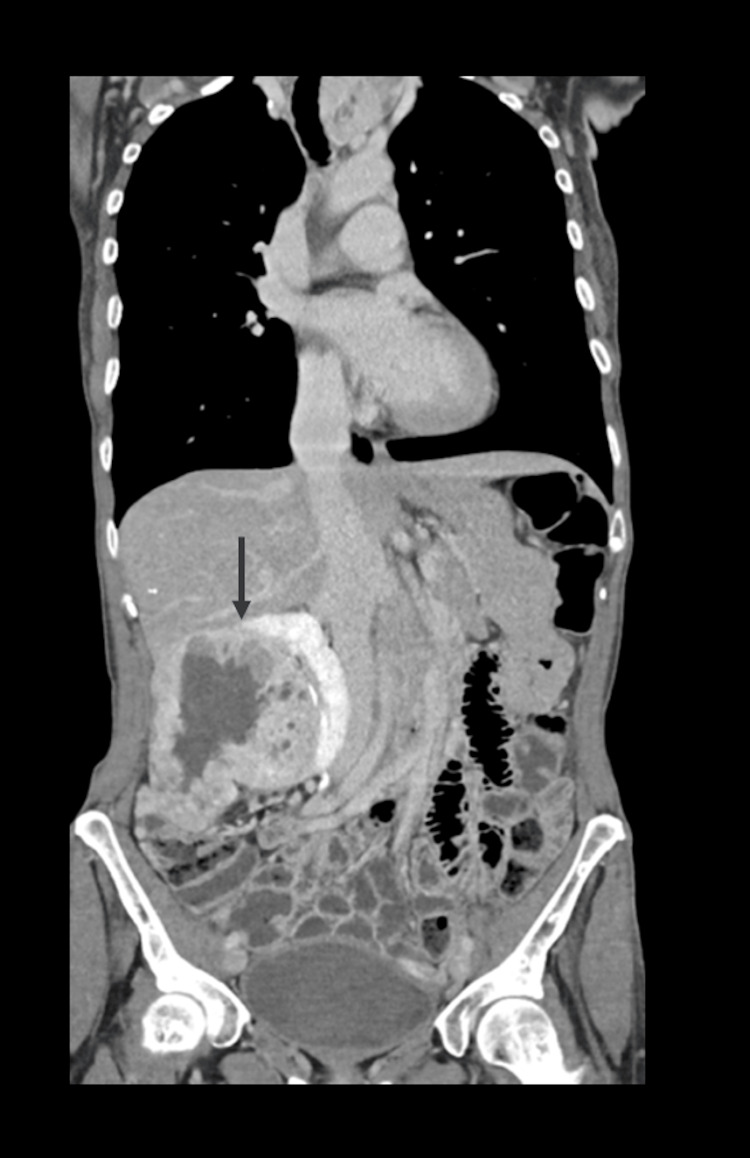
Contrast-enhanced abdominal CT showing a large heterogeneous right renal mass with necrosis and coarse calcifications.
